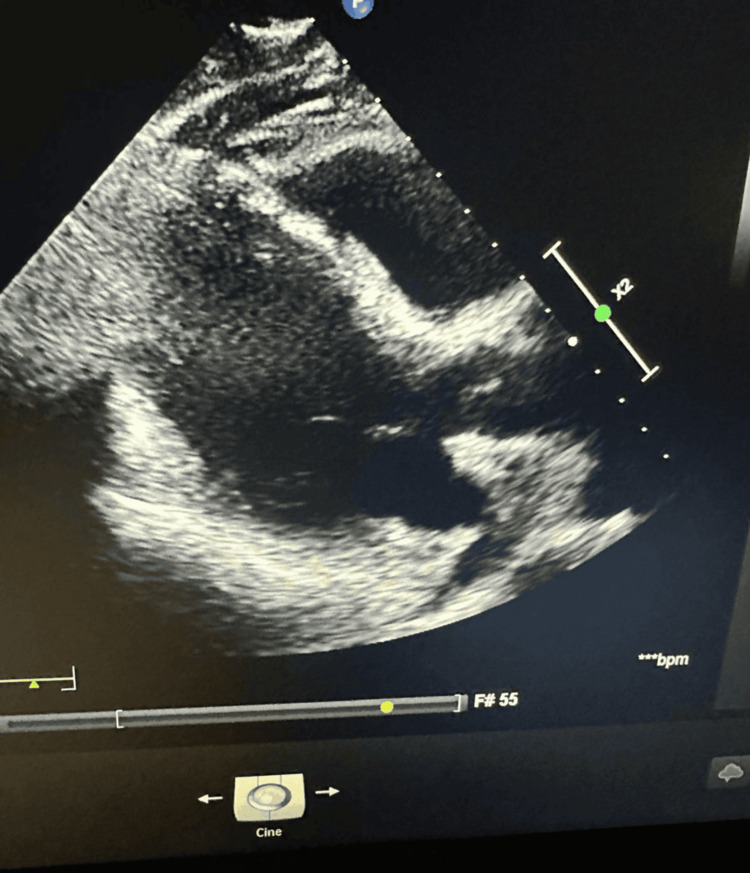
Transthoracic echocardiographic image demonstrating global hypokinesia with a left ventricular ejection fraction of 38% (Simpson’s biplane method)
